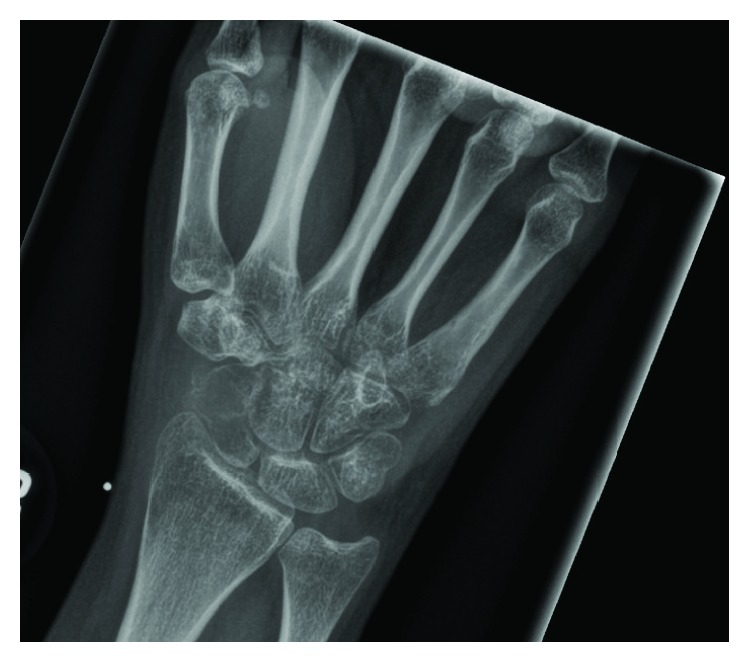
Diagnostic AP XR. AP radiograph of the right wrist, demonstrating radiolucency within the scaphoid.
